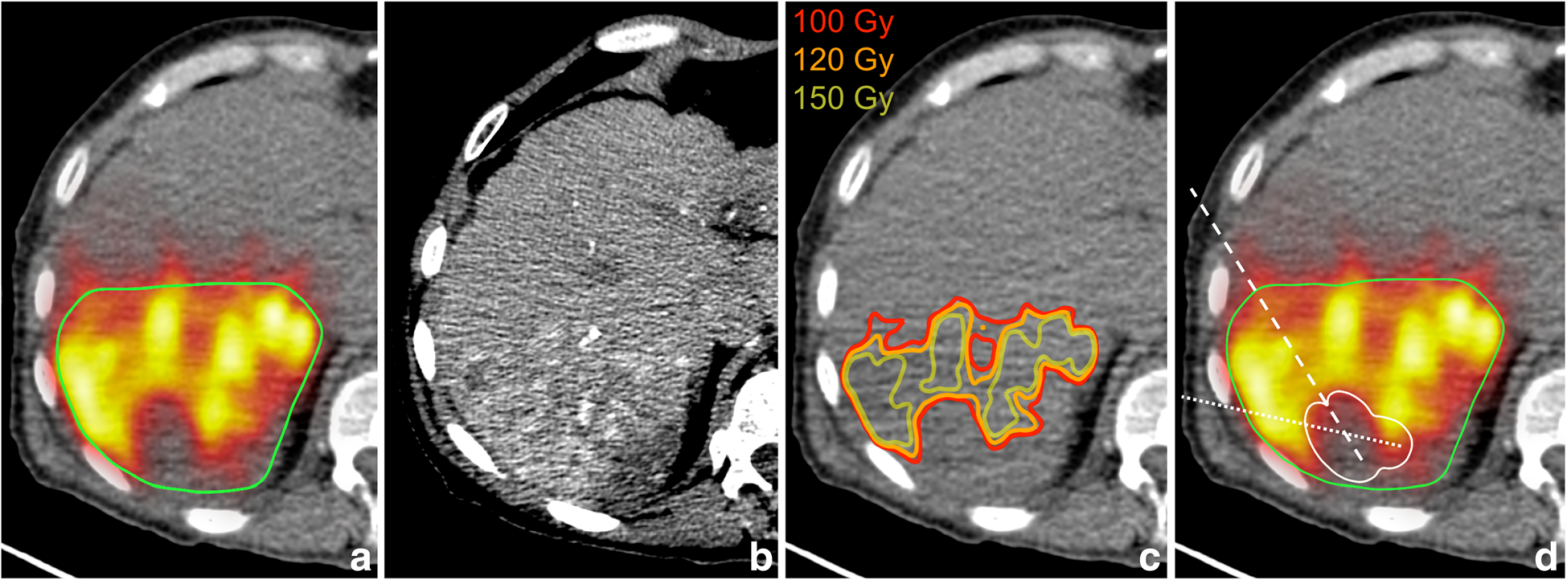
^90^Y PET/CT-guided treatment plan for Patient 5 (Table 1). a Post-radioembolization ^90^Y PET/CT with tumor contour (*green*). b Pre-treatment hepatic protocol CT. c 100-, 120-, and 150-Gy isodose curves computed from post-radioembolization ^90^Y PET/CT using the local deposition method. d Electrode placement in-plane (*dashed white line*), electrode placed 10 mm inferior to displayed slice (*dotted white line*) and RF ablation cell killing boundary (*solid white line*, Ω = 6.9)
